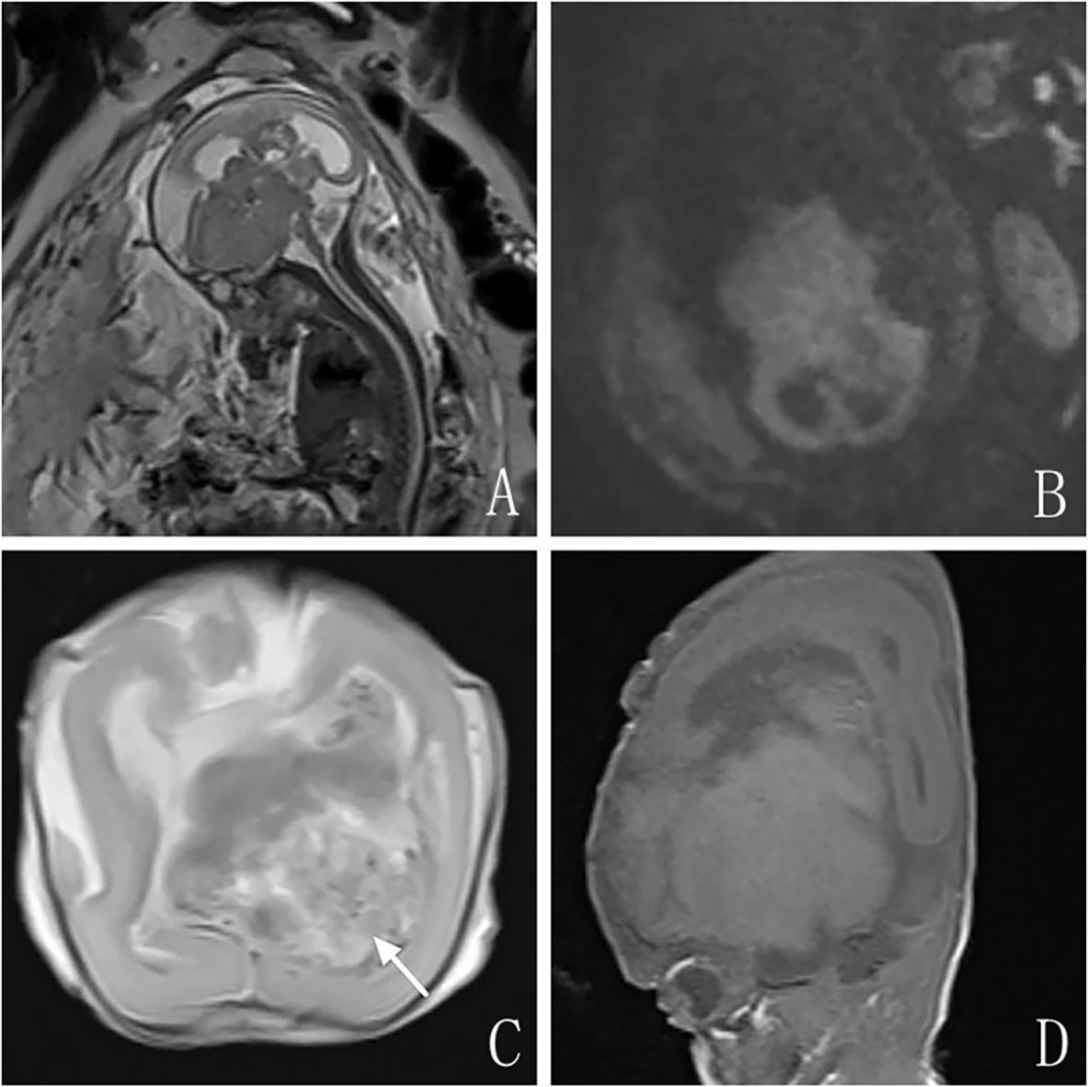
Prenatal and post-termination MRI findings. (A) Sagittal T2WI demonstrating the lesion at the anterior cranial base, closely related to the middle cranial fossa (long arrow), extending posteriorly from the midline, with slightly hyperintense signal. (B) Axial DWI (b = 700) showing the lesion with isointense signal, similar to normal brain parenchyma. (C) Post-termination axial T2WI showing the lesion protruding into the left lateral ventricle with heterogeneous signal intensity (long arrow). (D) Post-termination sagittal T1WI demonstrating posterior and superior displacement of normal brain parenchyma due to mass effect.
